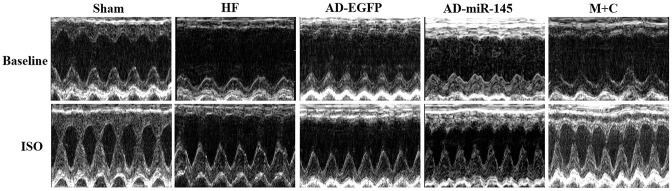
Representative images of M-mode echocardiography at baseline and 5min after ISO injection, respectively.
